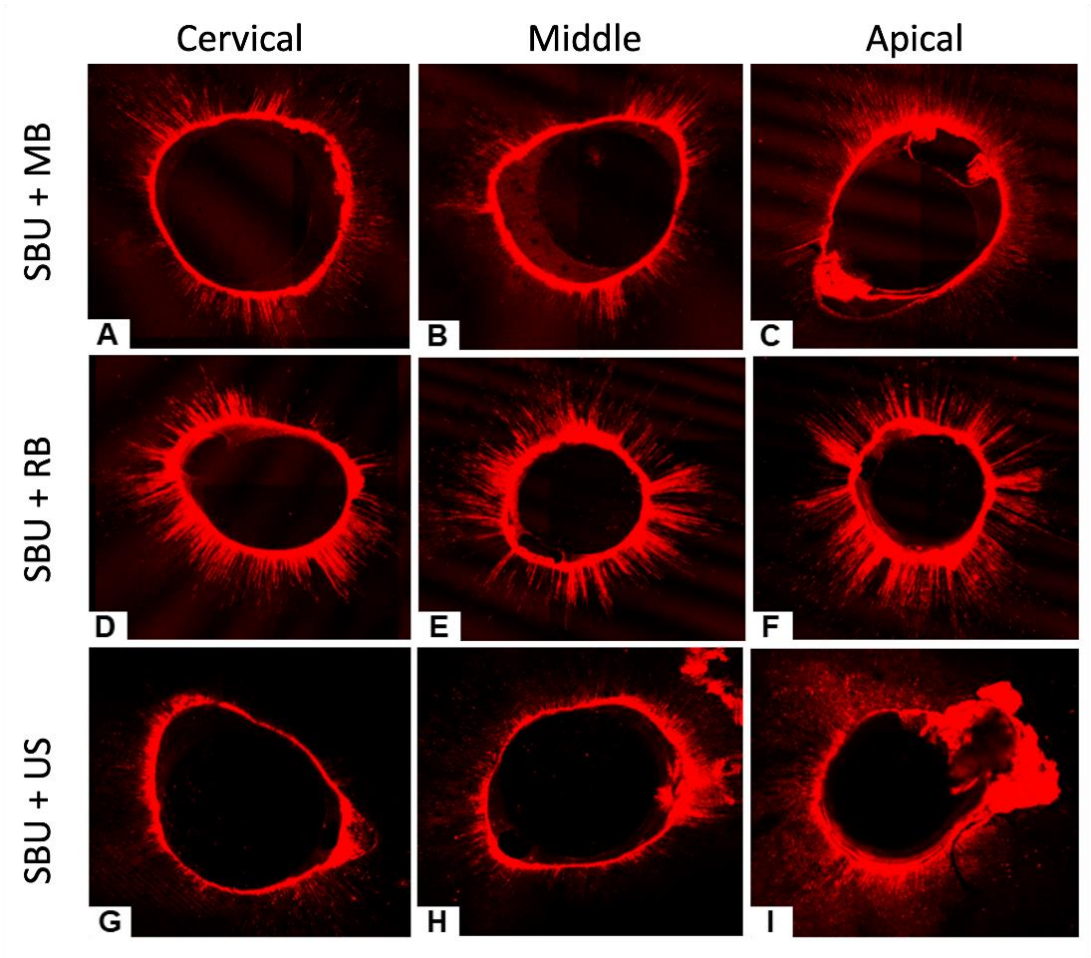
CLSM images showing the intratubular penetration of the Single Bond Universal (SBU). Single Bond Universal + microbrush cervical (A); middle (B); and apical third (C). Single Bond Universal + rotary brush cervical (D); middle (E); and apical third (F). Single Bond Universal + ultrasonic tip cervical (G); middle (H); and apical third (I).
